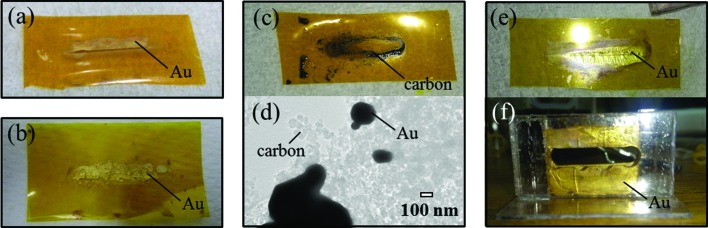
Photographs [(*a*)–(*c*), (*e*)–(*f*)] and TEM (*d*) image of metallic Au deposited *via* synchrotron X-ray irradiation: (*a*) PI film for sample 1, (*b*) PI film for sample 2, (*c*) PI film for sample 3, (*d*) carbon particles for sample 3, (*e*) PI film for sample 4, (*f*) acrylic cell for sample 4.
